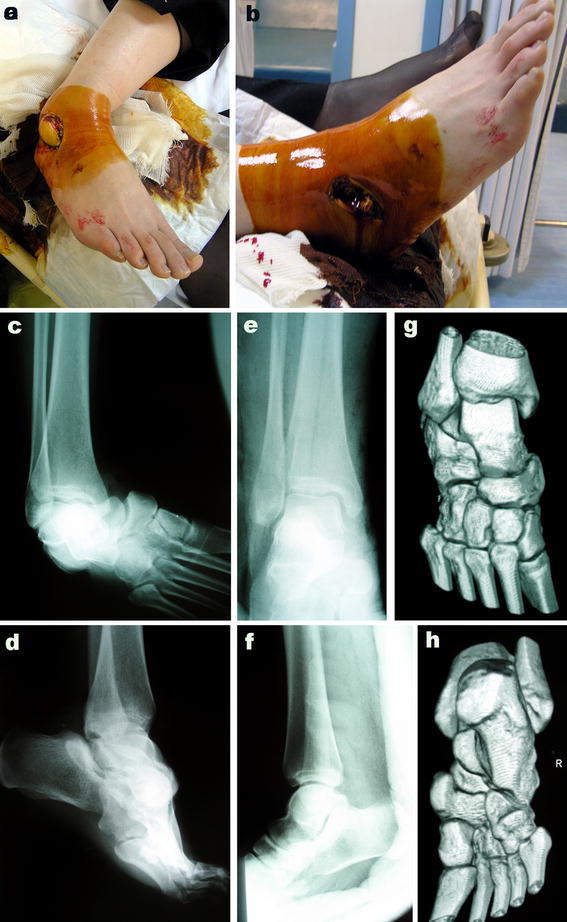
a, b Pre- and postreduction clinical views of the open medial subtalar dislocation of case 4. c–f Pre- and postreduction anteroposterior and lateral radiographs of the open medial subtalar dislocation of case 4. g, h Postreduction computed tomography with three-dimensional (3D) reconstruction views for the detection of any occult fracture
